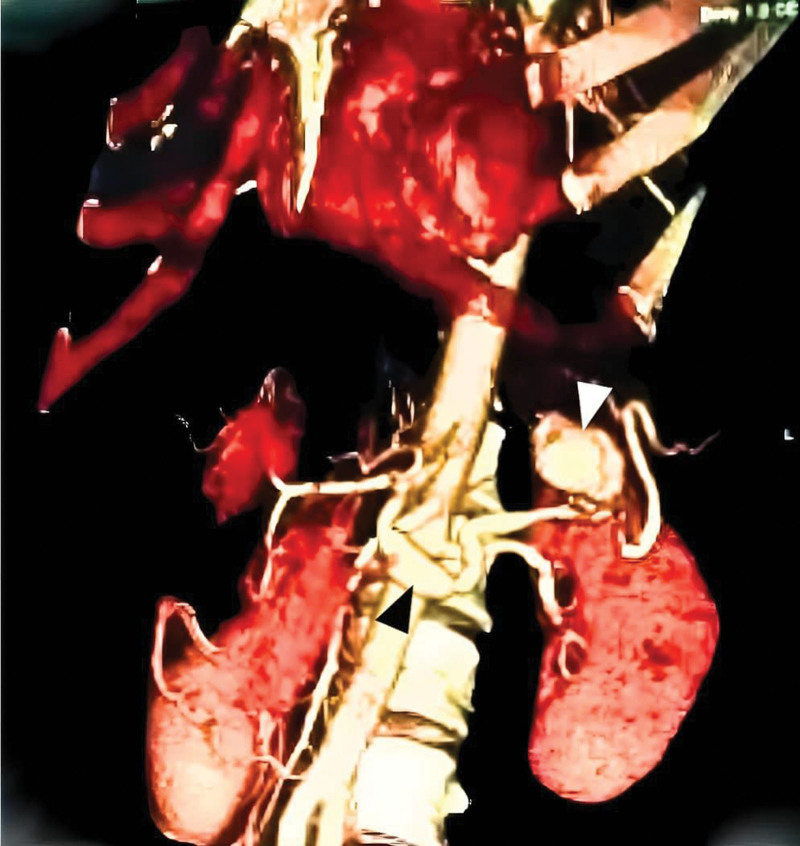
MSCT with three-dimensional reconstruction showing a SAA in the distal part of splenic artery (white arrow) which was done 7 months before current admission. Another aneurysmal dilatation in the first part of splenic artery is revealed (black arrow). MSCT = multi-slice computed tomography, SAA = splenic artery aneurysm.
